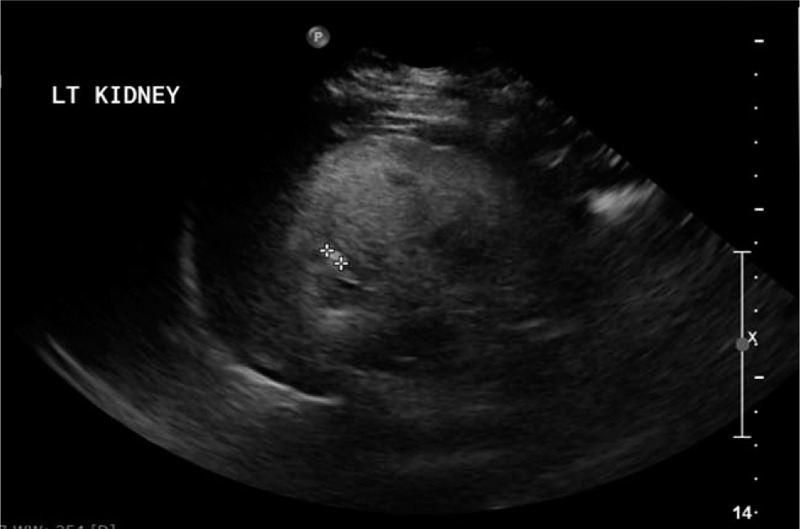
Ultrasound of left kidney obtained in sagittal view demonstrates stone measuring about 5 mm.
